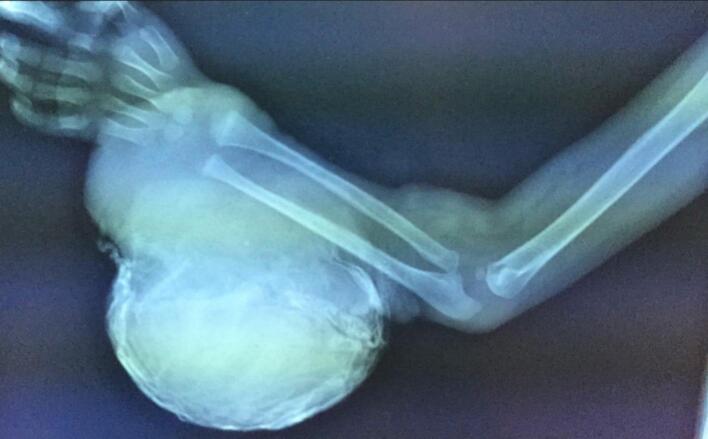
Plain radiography, lateral projection of the right upper extremity, tumor is observed in the right forearm, does not involve the humerus or radius, increased volume of soft tissues.
